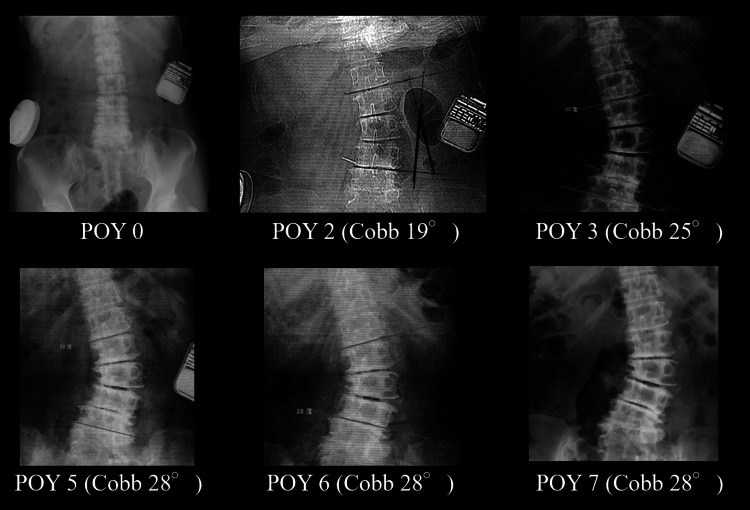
X-rays before and after ITB therapy. Compared to the X-ray performed in POY zero, scoliosis was evident in POY two. Following the removal of the ITB pump in POY five, decompression surgery was electively performed in POY six. Thereafter, the progression of scoliosis ceased for two years and Cobb angle remained at 28 degrees. The rectangular object in the left abdomen is an implantable pulse generator for motor cortex stimulation. ITB: intrathecal baclofen; POY: postoperative year
